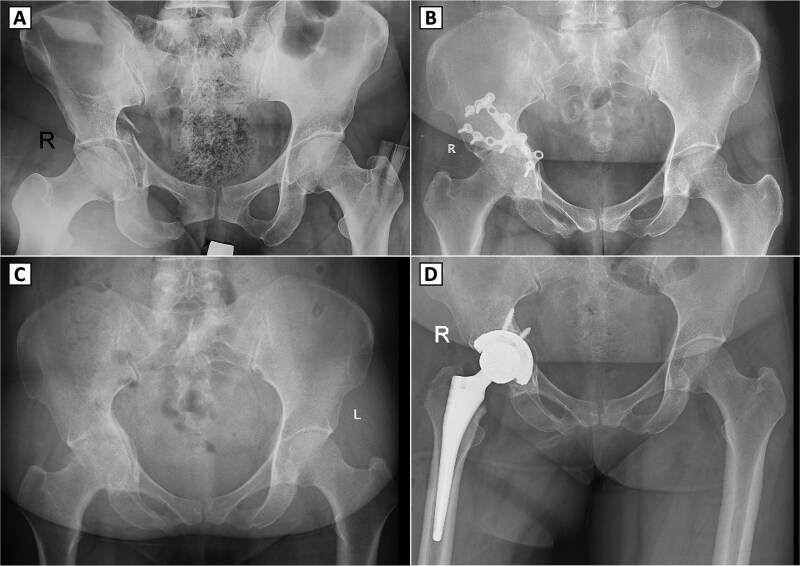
Serial radiographs of a patient with right acetabular fracture treated with open reduction and internal fixation (ORIF), followed by secondary osteoarthritis and conversion to total hip arthroplasty. (A) Preoperative anteroposterior (AP) pelvic X-ray showing a displaced right acetabular fracture. (B) Postoperative AP radiograph demonstrating internal fixation with reconstruction plates and screws on the right acetabulum. (C) Follow-up AP pelvic radiograph showing signs of secondary osteoarthritis in the right hip joint, showing shows signs of secondary osteoarthritis in the right hip joint, including joint space narrowing, femoral head deformity, and subchondral sclerosis. (D) Postoperative AP radiograph after conversion total hip arthroplasty with a cementless prosthesis and screw fixation.
